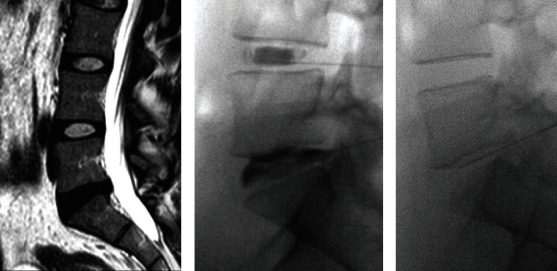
MRI showing disc desiccation at L5/S1 (a); discography showing abnormal dye pattern at L5/S1 (provoked concordant low back pain) and normal dye pattern at L4/5 (no pain provocation) (b); intradiscal BMAC injection at L5/S1 (c).
